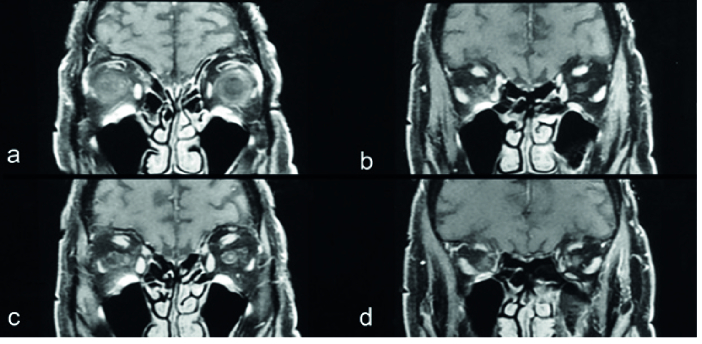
Coronal T1-weighted brain MRI scans with gadolinium administration showing mild optic nerve enhancement, visualizing no brain lesions or intraocular tumors.
